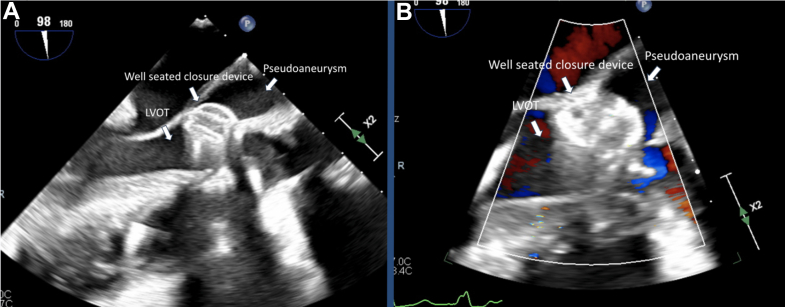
Transesophageal Echocardiography After Paravalvular Leak Closure (A) Transesophageal echocardiography shows the vascular plug preventing flow from the pseudoaneurysm into the LVOT, and (B) color Doppler shows no flow from the pseudoaneurysm into the LVOT. LVOT = left ventricular outflow tract.
